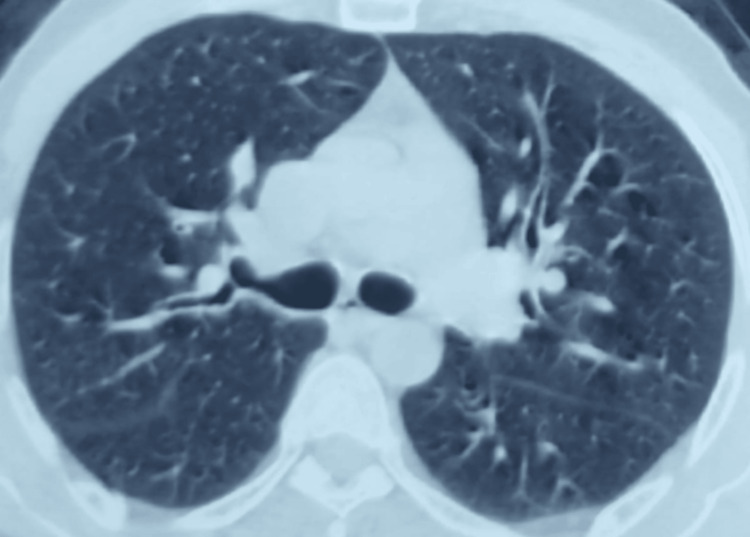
Representative axial chest CT images obtained after three months of corticosteroid therapy demonstrating near-complete radiological regression of the previously described lesions CT: computed tomography
